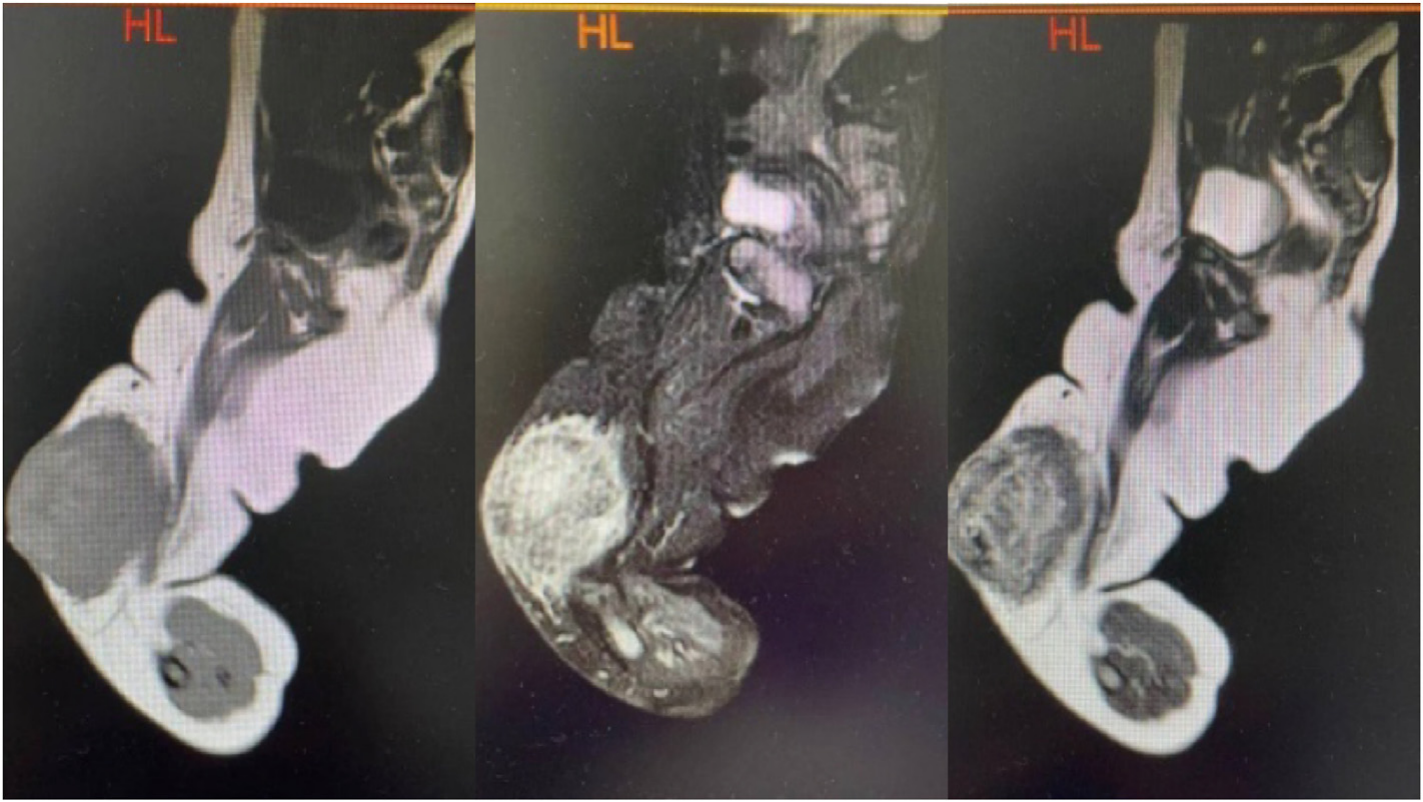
The enhanced MRI scan of the infant's right lower limb.
